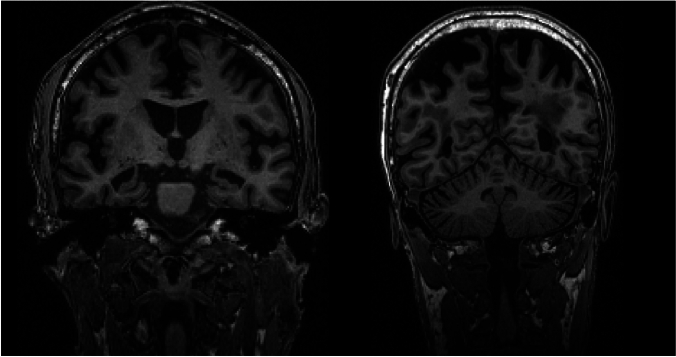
Coronal 3D T1-weighted brain MRI scans with MTA = 3 bilaterally (left panel) and with GCA-P = 3 (right panel)
